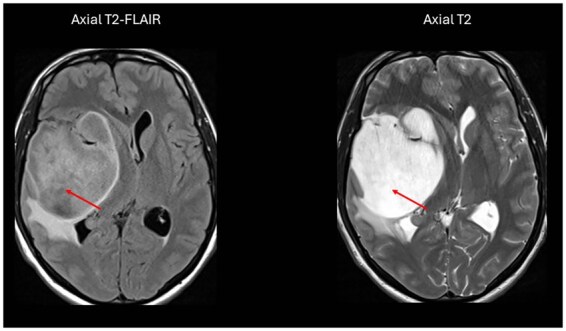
T2/FLAIR mismatch sign is visible in an *IDH-*mutant astrocytoma.
